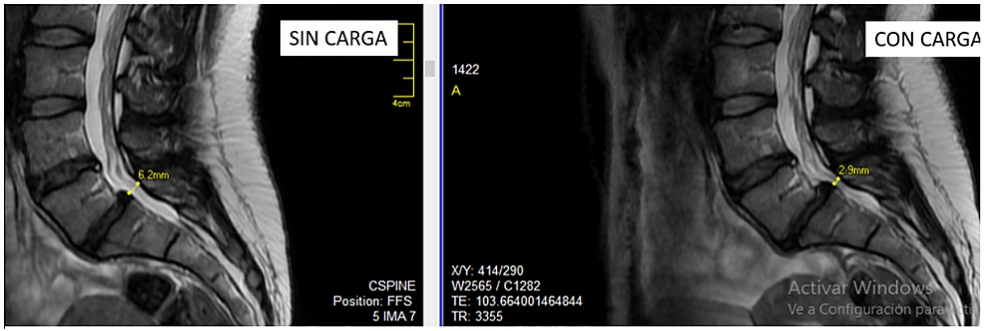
Images showing the same patient with a herniated disc at L5-S1 but the channel diameter is reduced by more than half with a load than without (6.2 mm on left, 2.9 mm on right). Axial loading is not represented when the patients reclined during the MRI MRI, magnetic resonance imaging.
